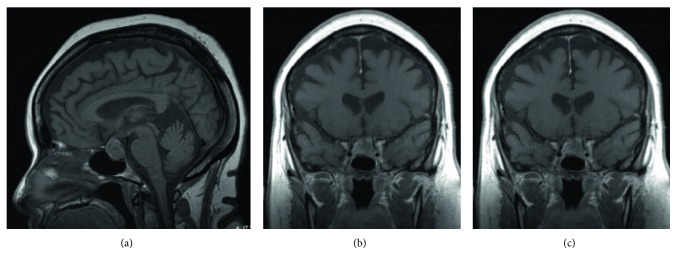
MRI head with contrast T1. (a) Sagittal view showing an enlarged pituitary gland. It measures 1.9 × 2.0 cm in the greatest dimension. The mass is slightly heterogeneous in signal and is slightly impressing on the optic chiasm. (b) and (c) Coronal view with different cuts showing extension on the left cavernous sinus.
